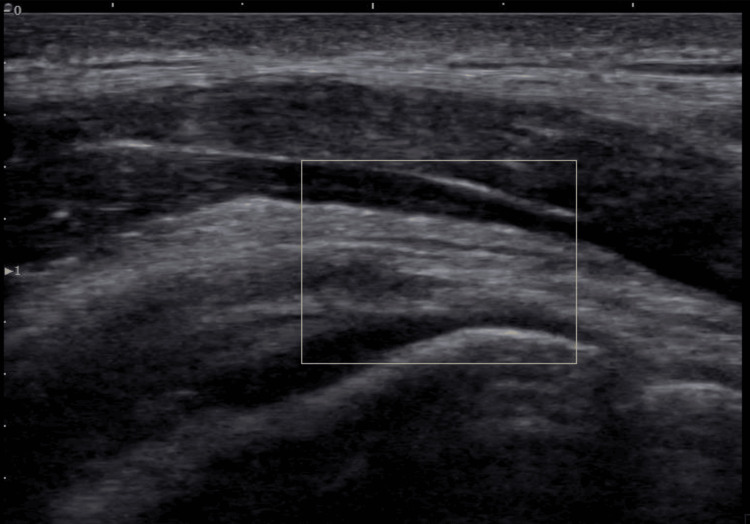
Second pre-treatment ultrasound view of the right anterior supraspinatus
